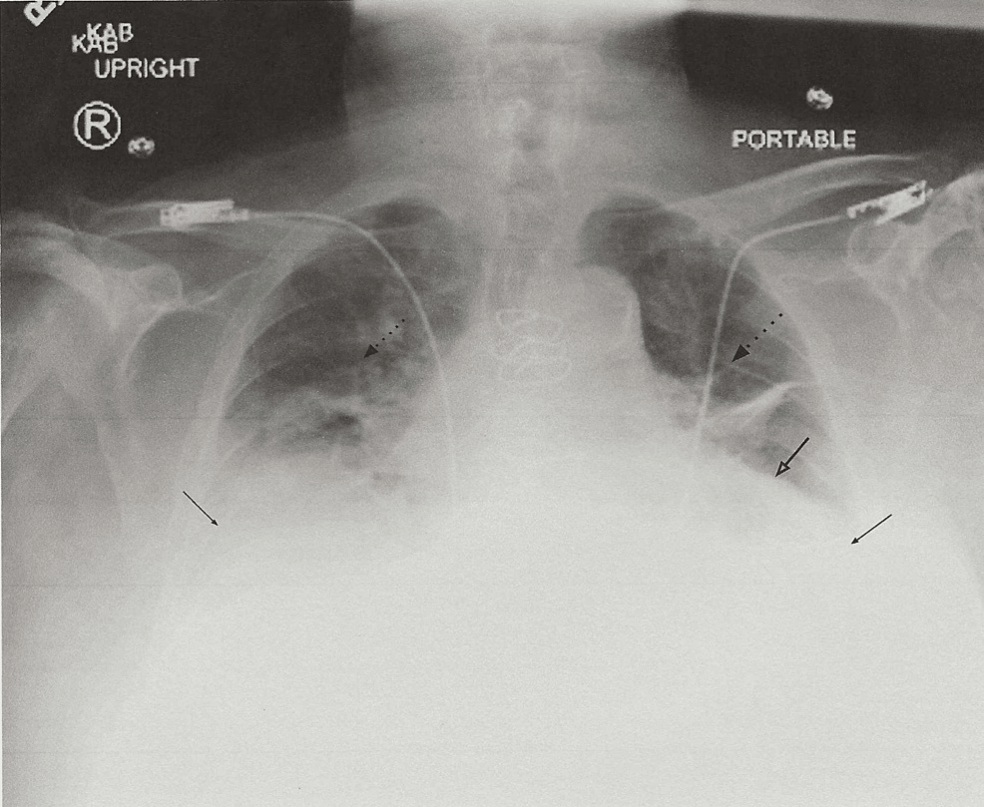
Chest X-ray showing cardiomegaly (arrow with open head), interstitial opacities (broken arrow), and bilateral pleural effusions (solid arrow with closed head)
